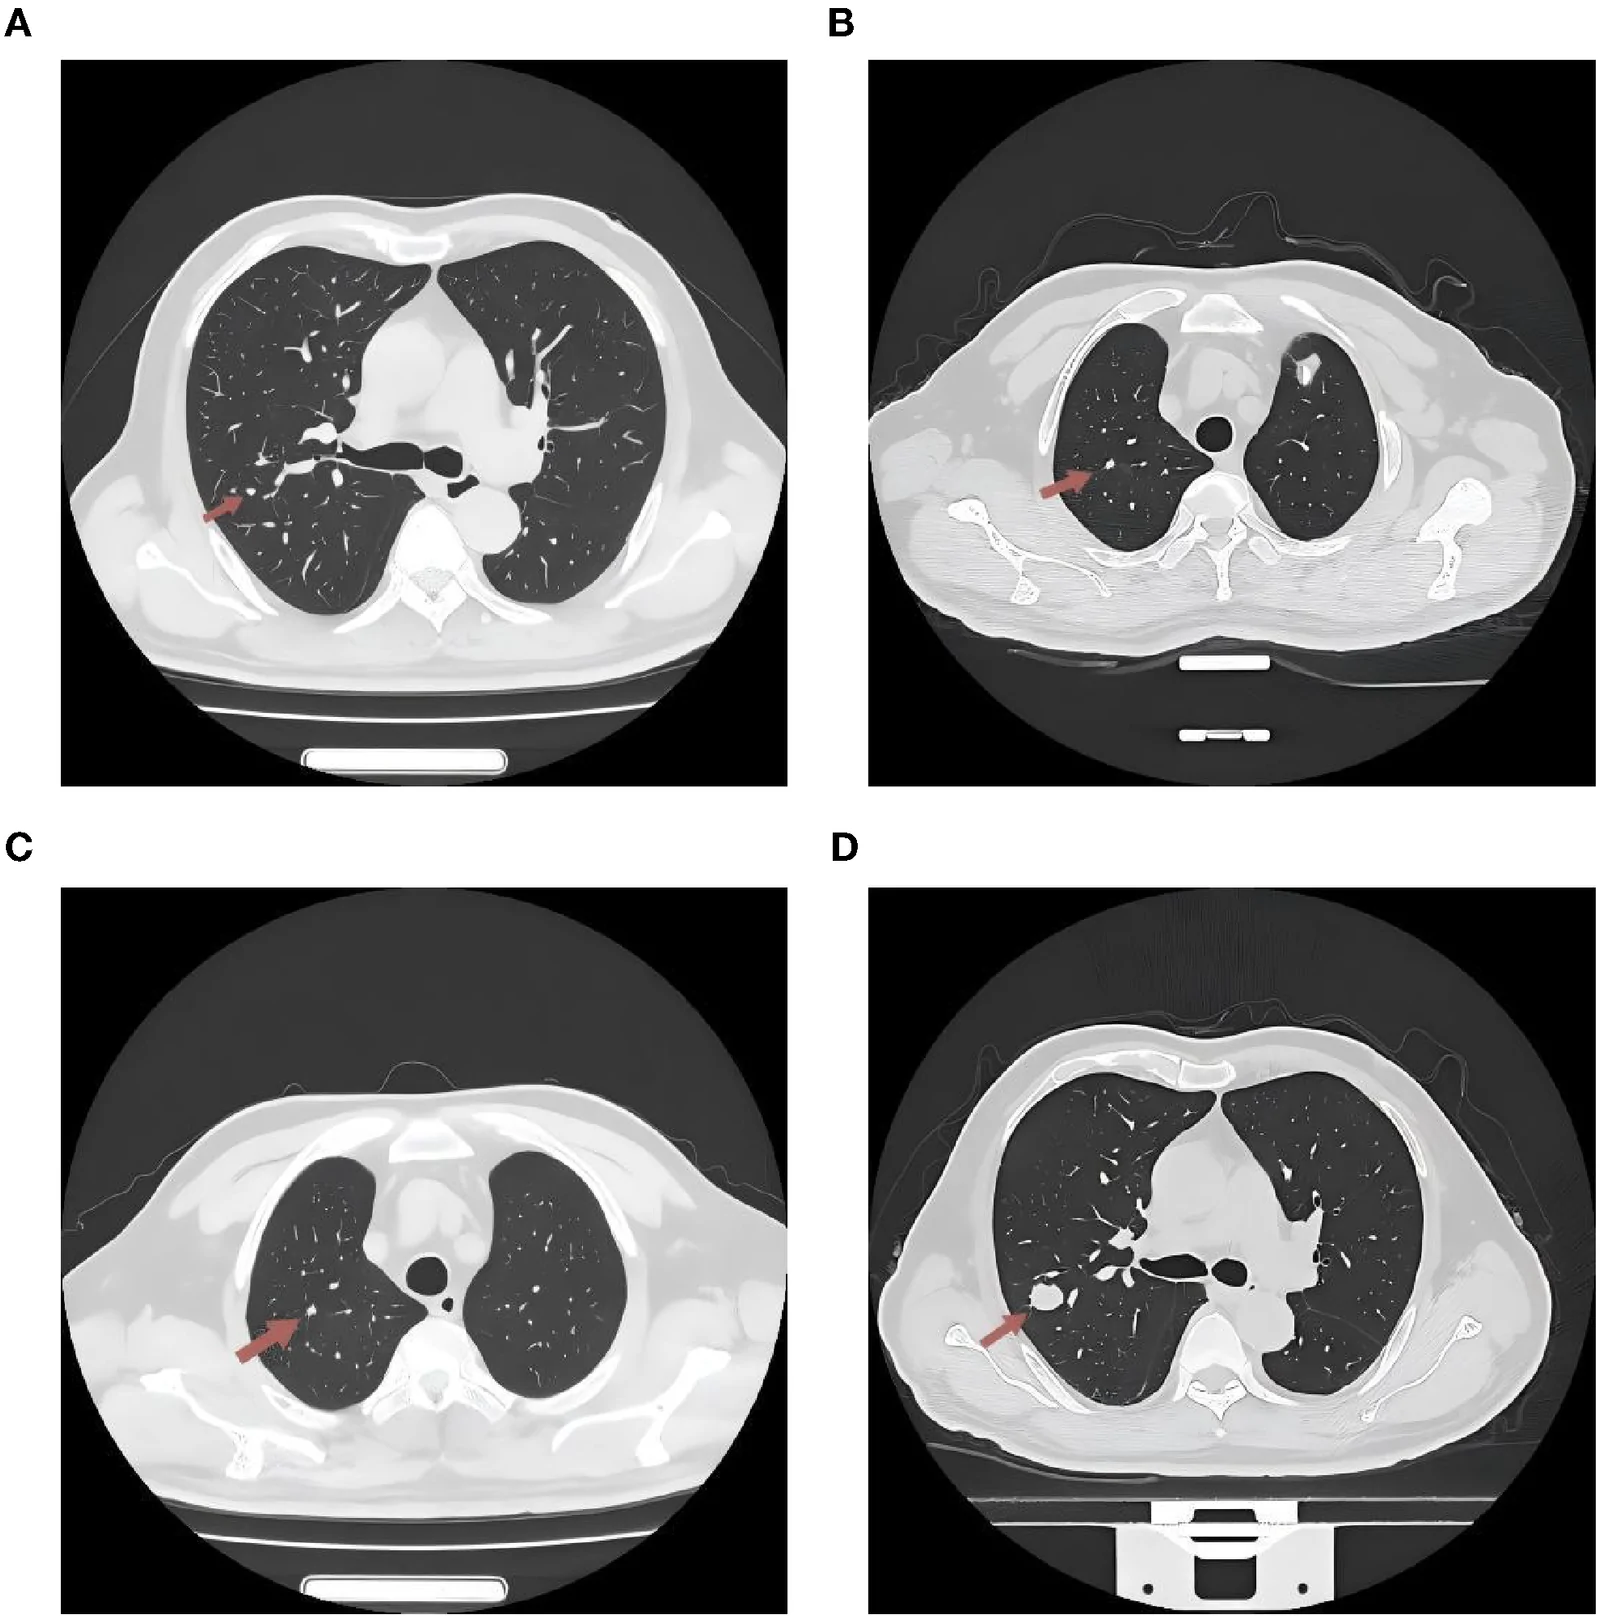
(A) January 2024 CT image showing a small solid nodule in the posterior segment of the right upper lobe (red arrow). (B) February 2025 CT image showing a stable nodule in the apical segment of the right upper lobe (red arrow). The faint opacity visible in the left upper lobe in this image is an artifact caused by respiratory motion, confirmed on adjacent CT slices which showed no corresponding parenchymal lesion. (C) January 2024 CT image showing the nodule in the apical segment of the right upper lobe (red arrow). (D) February 2025 CT image showing the enlarged lesion in the posterior segment of the right upper lobe, measuring 1.7 cm × 1.5 cm, with lobulation sign (red arrow). The posterior segment nodule was confirmed as SCLC (panels A and D); the apical segment nodule was confirmed as MIA (panels C and B).
